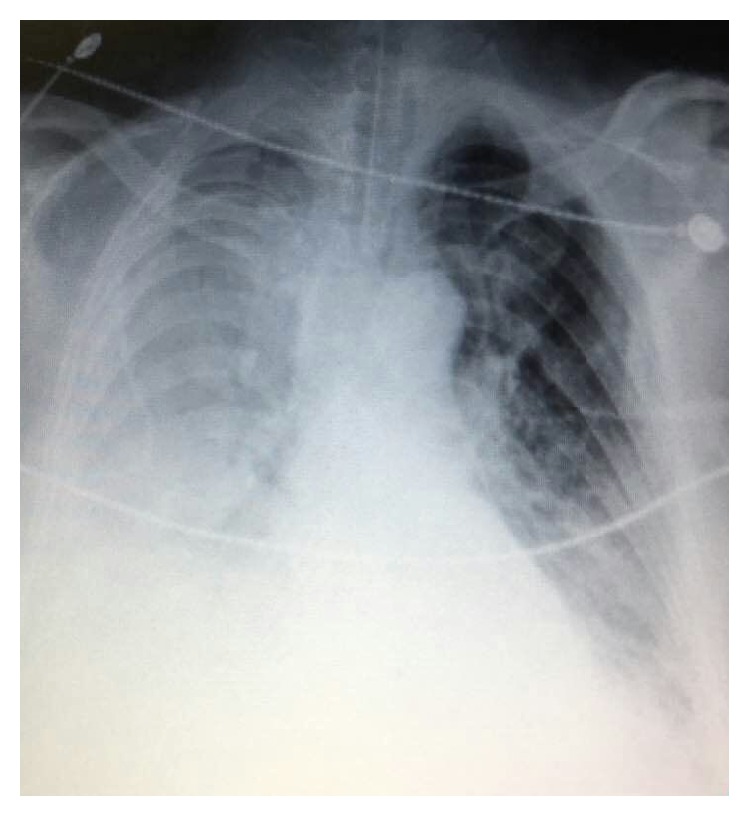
Chest X-ray exam of case 2. Large hyperdensity in right lung showing right lung consolidation, which represented right lung pneumonia in this case.
